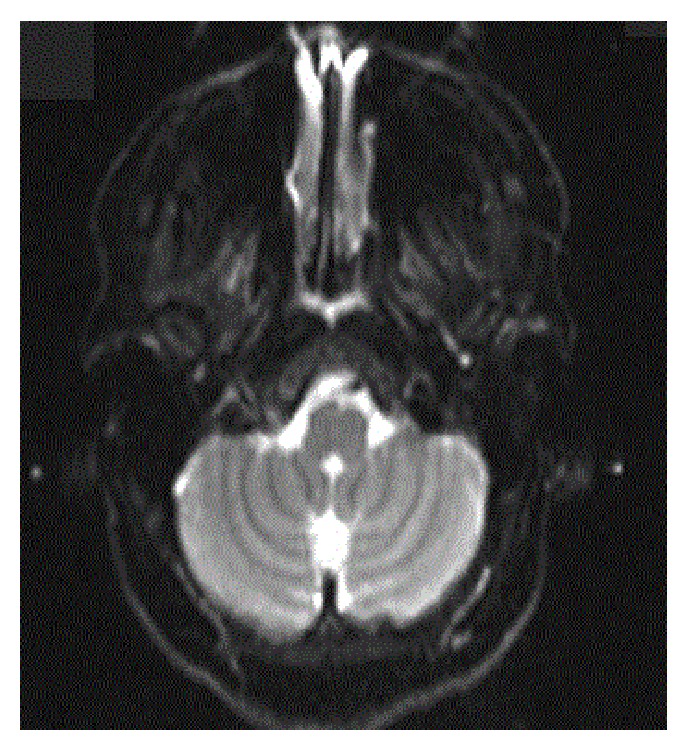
MRI of head and neck at 3-month follow-up visit.
